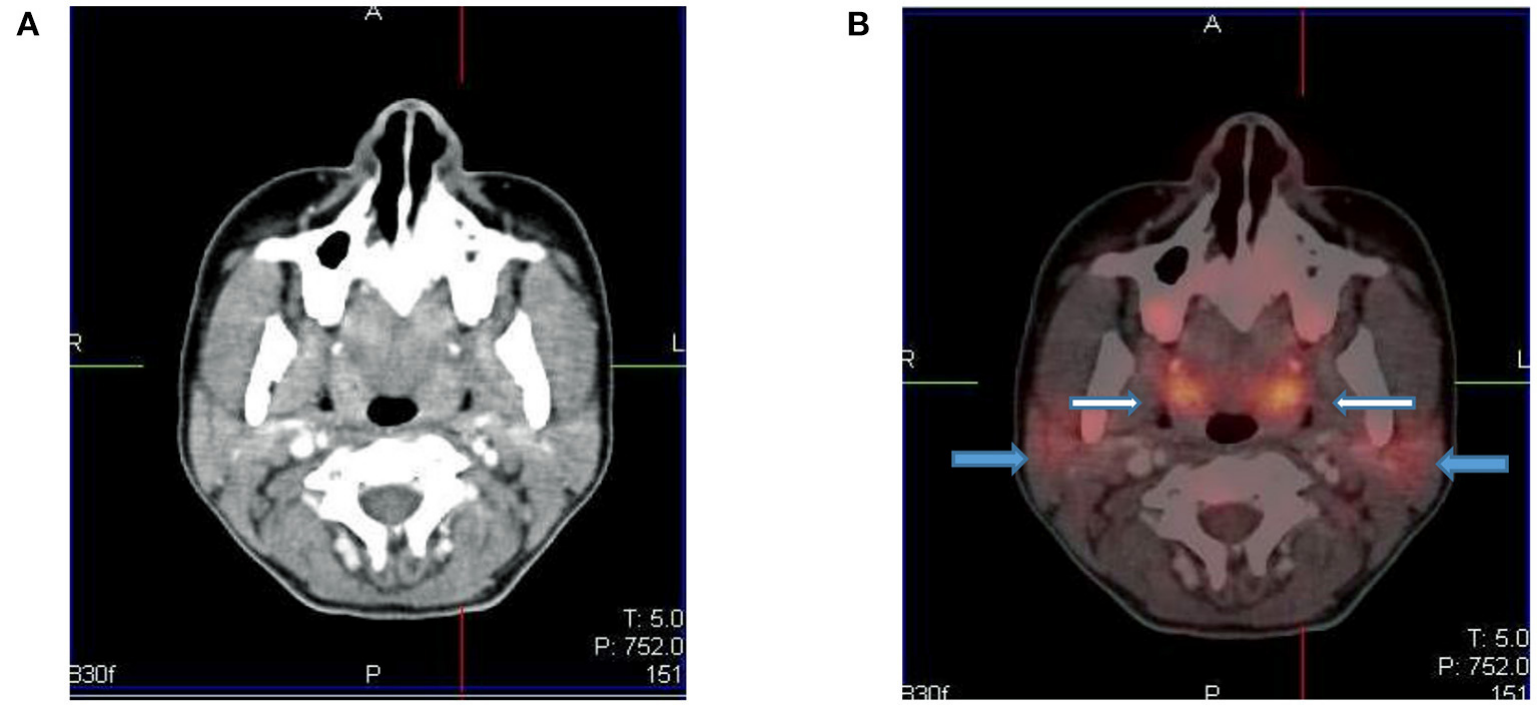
Axial CT (A) and fused PET/CT (B) images showing symmetric increased uptake in the tonsils (white arrows) and bilateral mild uptake in the parotid glands (blue arrows).
